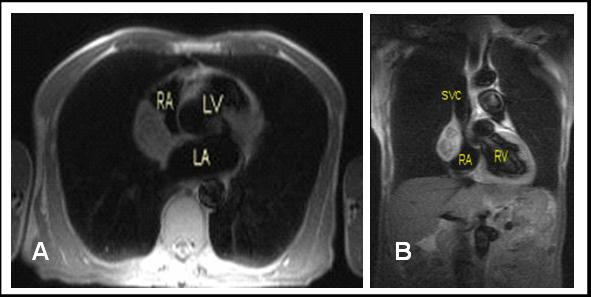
MRI heart. A. Sagittal. B. Coronal. RA = Right atrium, LA = Left atrium, LV = Left ventricle, SVC = Superior vena cava. The close proximity of the tumor to the right atrium and extension towards a thickened interatrial septum can be seen.
